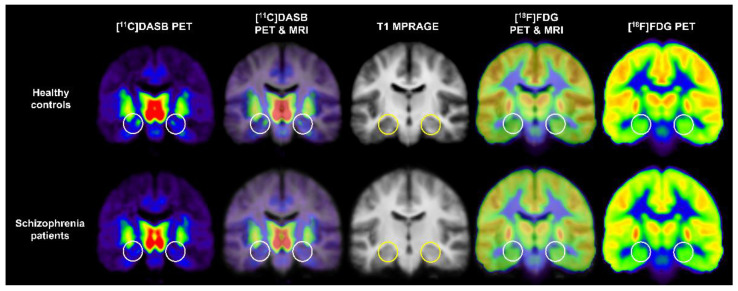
Mean [^11^C]DASB PET, [^18^F]FDG PET, T1 MRI, and PET–MRI fusion images of healthy controls and patients with schizophrenia. Circles indicate the location of the bilateral anterior hippocampus.
